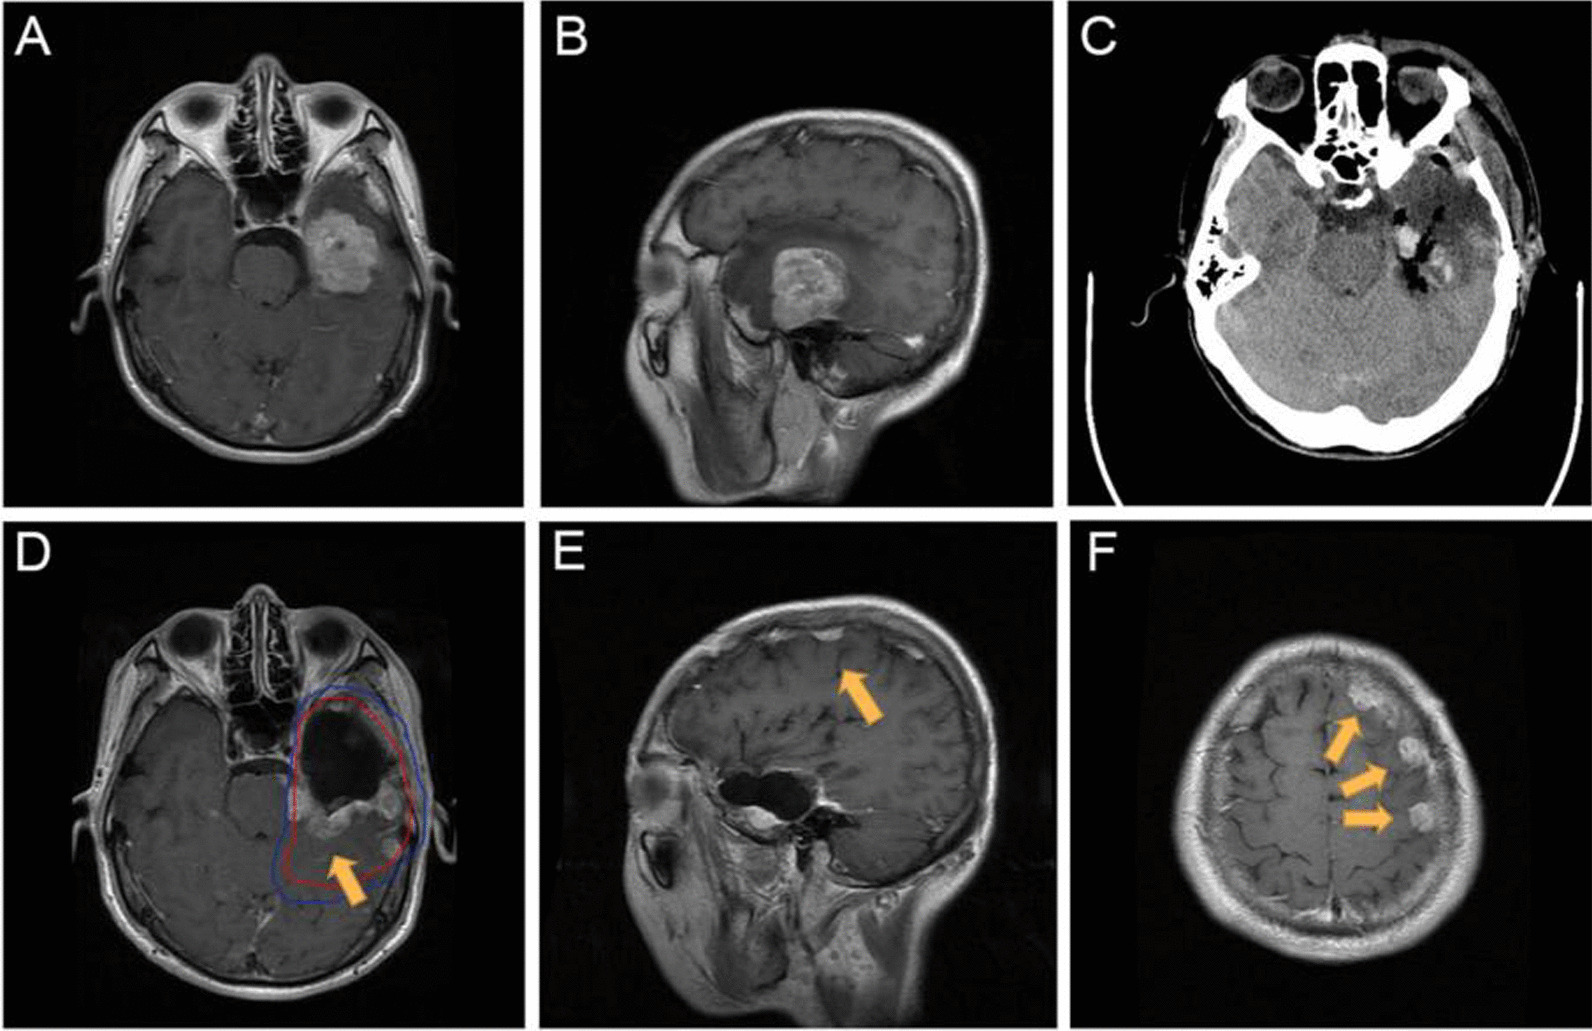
MRI scans from a patient who developed representative dissemination recurrence. A Preoperative axial enhanced T1-weighted images and B preoperative sagittal enhanced T1-weighted images showed that the tumor was in the left frontotemporal lobe. C CT images 1 day after operation. D–F Enhanced T1-weighted images follow-up 6 months after resection showed a new in-field lesion and multiple meningeal metastasis (yellow arrows). The blue and red lines represent the isodose lines of PTV, CTV, respectively. *MRI* Magnetic resonance imaging
